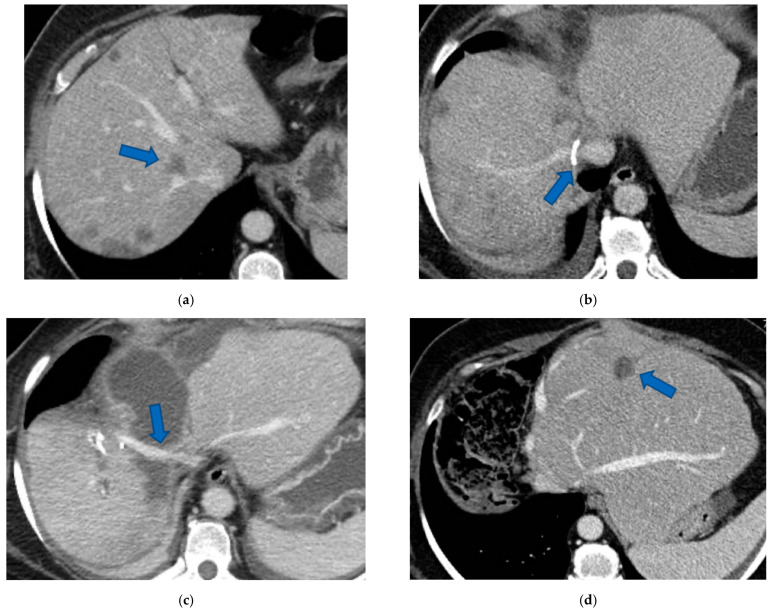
CT Images of staged e-ALPPS performed to treat 55 bilateral CRLMs infiltrating RHV and MHV. (a) Partial response after FOLFOXIRI + Panitumumab 8 cycles; FLR (=part of S2/S3) volume is 7%; blue arrow indicates CRLM infiltrating RHV and MHV at the hepatocaval confluence. (b) Seventeen days after the first stage (resection of S1/S4 *en-bloc* with MHV and extended to S2/S3; RHV is suspended on a vessel loop; FLR (=part of S2/S3) volume increases up to 28% and an intermediate surgical stage for partial resection of deportalized liver is planned to boost liver regeneration; blue arrow indicates elastic vessel loop around RHV. (c) Seven days after intermediate stage (resection of S5/S8 *en-bloc* with RHV reconstructed with end-to-end anastomosis) FLR (=part of S2/S3) volume increases up to 41% and the third stage is planned to complete the staged e-ALPPS; blue arrow indicates reconstructed RHV. (d) Nineteen months after staged e-ALPPS the patient is disease free; a CRLM was treated by percutaneous ablation (blue arrow) 5 months after staged e-ALPPS. Abbreviations: e-ALPPS: enhanced ALPPS; CRLM: colorectal liver metastases; RHV: right hepatic vein; MHV: middle hepatic vein; FLR: future liver remnant; S: segment.
